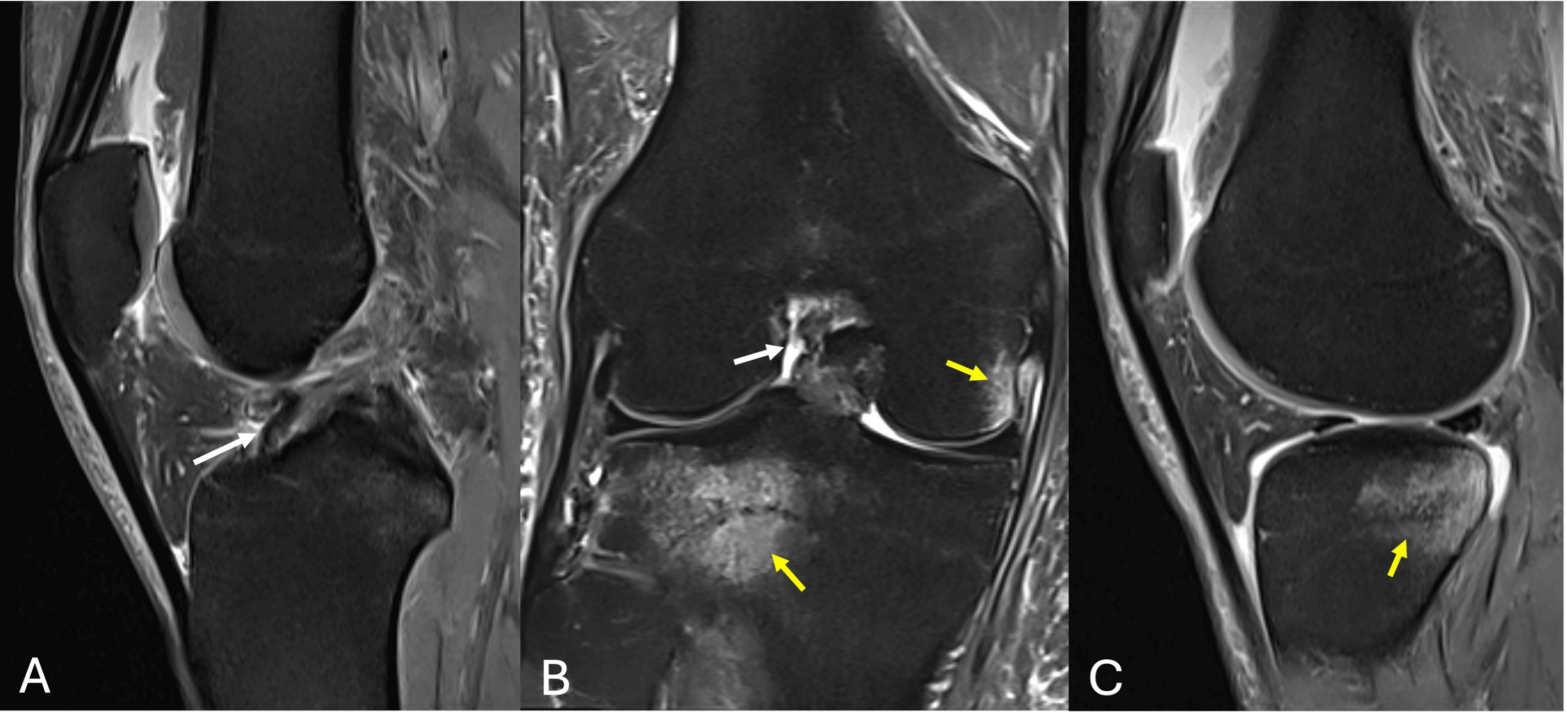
PDFS MRI (A) sagittal and (B) coronal images showing complete ACL tear from its femoral attachment (white arrows). PDFS MRI (B) coronal and (C) sagittal images showing bony contusions involving the posterolateral tibial plateau and lateral aspect of medial femoral condyle PDFS : proton density fat-saturated; ACL: anterior cruciate ligament
